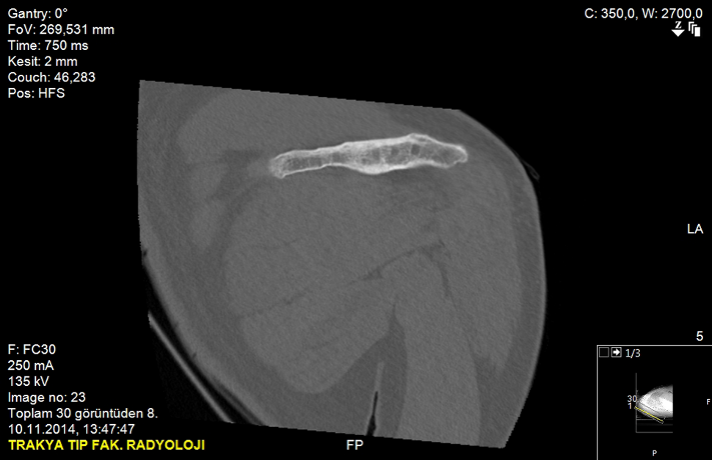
Follow-up computed tomography coronal section
